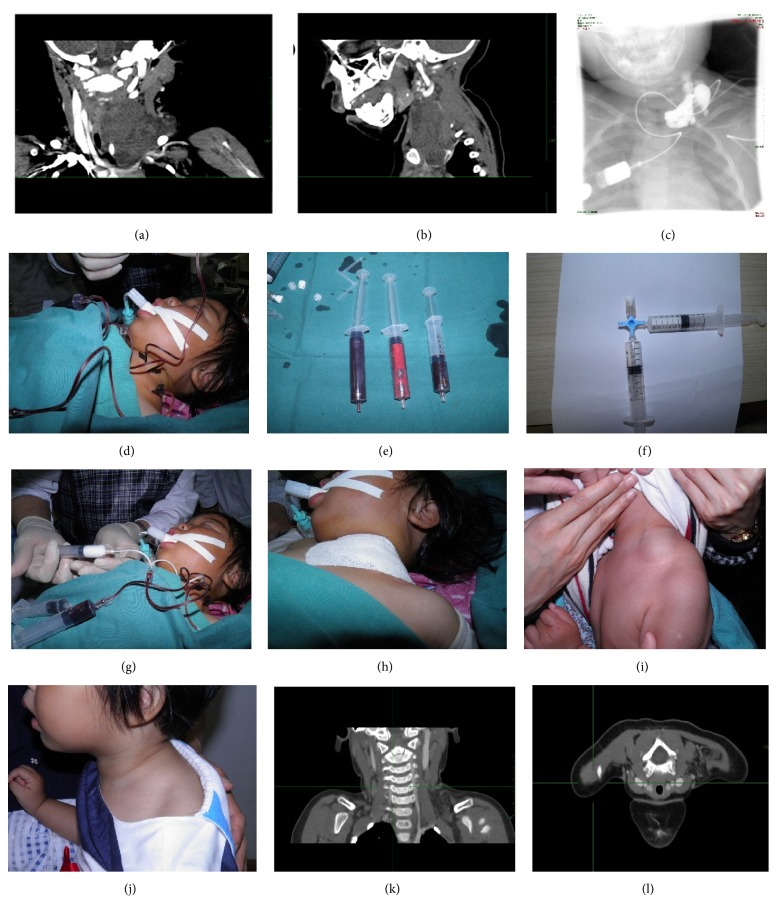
17-month-old female with lymphatic malformations (LMs) in the left cervical region that suddenly expanded over a 10-day period. The heart rate decreased and she underwent endotracheal intubation for dyspnea. (a)-(b) Computed tomography (CT) image revealing multilocular LMs (58 × 37 × 50 mm). The airway is obviously compressed and shifted. (c) Imaging for positioning. (d)-(e) Multiple points were percutaneously punctured and 25 mL of fluid was aspirated. (f) Preparation for the lauromacrogol foam injection. (g) Injection of the lauromacrogol foam. (h) The local compression bandage applied after treatment. (i) At the 1-month follow-up appointment, the mass was obviously shrunken. Three additional treatment sessions were then completed. (j) After 6 months, the mass disappeared. (k)-(l) The CT scan showing that the cyst was obviously decreased and the residual cystic cavity showed no lymph left.
